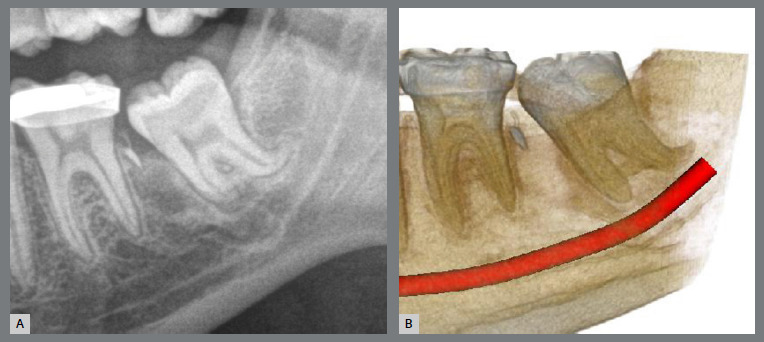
Close-up images of the outcome of molar traction: A) panoramic radiograph close-up; and B) cone-beam computed tomography close-up.
